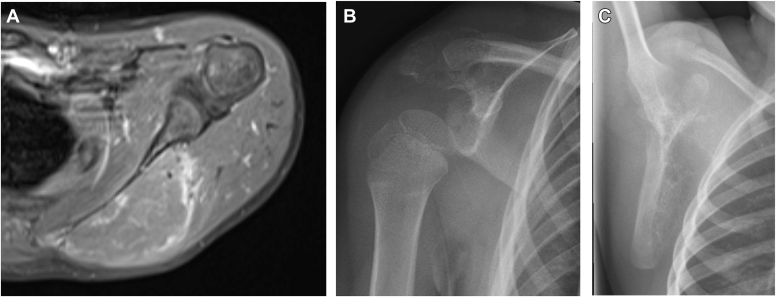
Initial imaging of the scapula tumor of patient 1-3. (A) Saggital magnetic resonance imaging (MRI) scan showing the Ewing sarcoma of the scapula body of patient 1. (B) Radiograph demonstrating the acromial Ewing sarcoma of patient 2. (C) Radiograph showing the Ewing sarcoma of the scapula body of patient 3.
